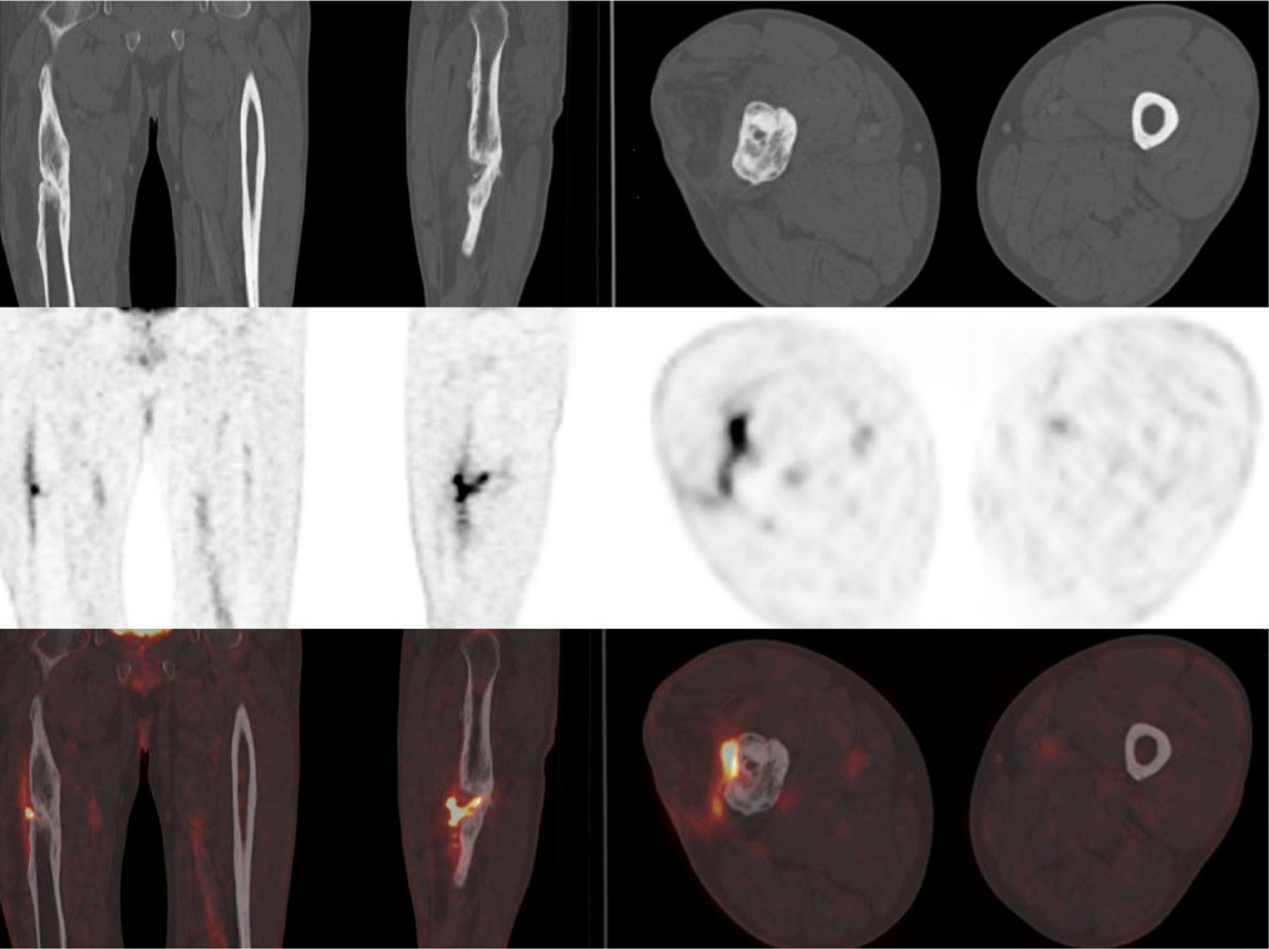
55-year-old patient with oligotrophic non-union. No complete osseous consolidation was seen for 6 months. Pathological, irregularly distributed [^18^F]FDG uptake (SUV_max_ 7.7, SUV_ratio_ 7.4) was found along the bone–bone interface, with a clear “hotspot” along the lateral fissure of the femur. The elevated [^18^F]FDG uptake extends to the proximate soft tissue. Atrophy of the *M. vastus lateralis* is seen due to immobilization. The [^18^F]FDG PET/CT scan was rated true positive in all image analysis based on positive intraoperative microbial tissue culture
